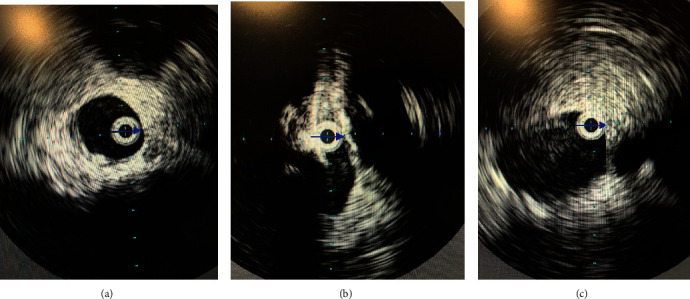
Intravascular ultrasound (IVUS) of the iliac vein: (a) precompression; (b) site of compression; (c) postcompression.
